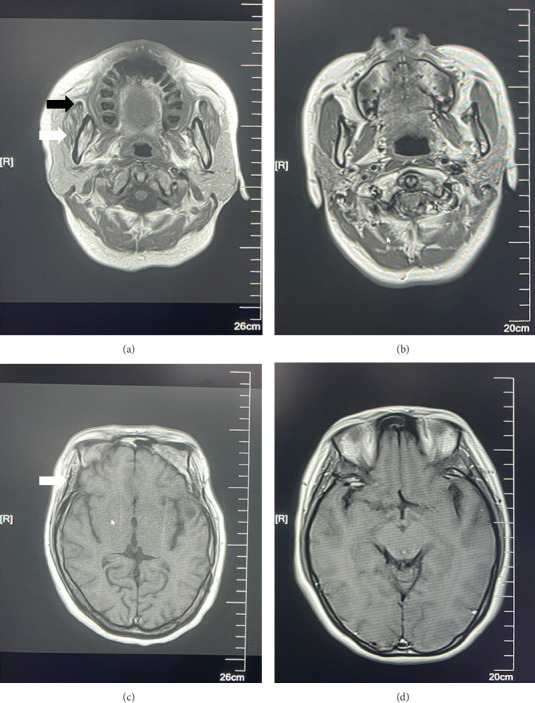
MRI scans of the masseter and temporal muscle of the MMTAH patient. (a) MMTAH masseter; (b) normal masseter muscle; (c) MMTAH temporal muscle; and (d) normal temporal muscle. The white arrows indicate muscles, and the black arrow indicates tendons. In contrast to normal, white fat infiltration and hyperplasia of the masseter tendon were observed in the temporal muscle of the MMTAH masseter.
